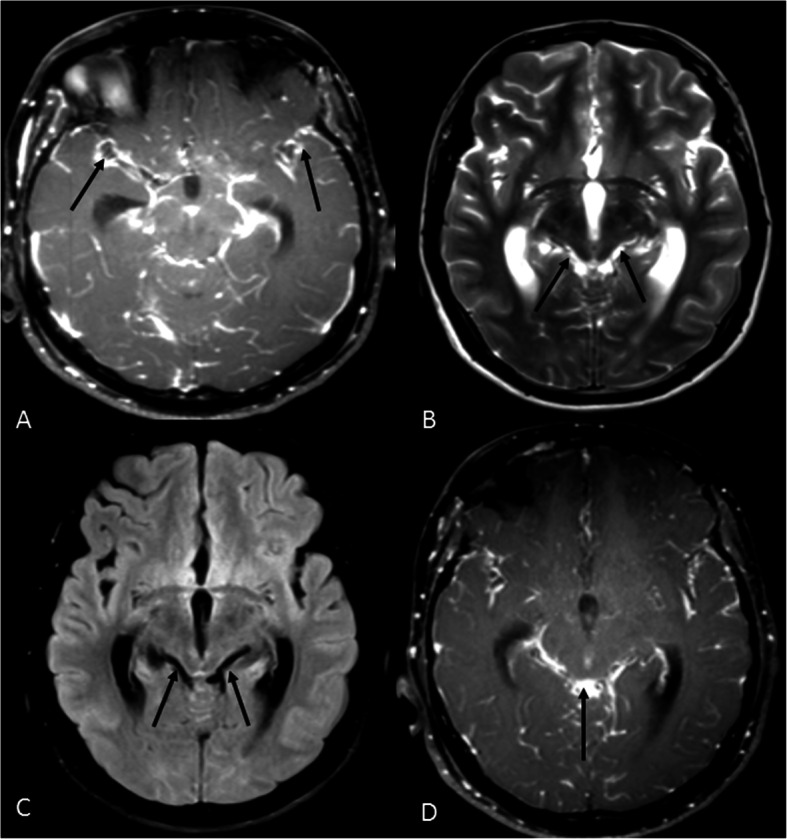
Magnetic resonance imaging of the brain at 2 months following the onset of symptoms. A Axial T1W post-gadolinium image of the brain at the level of the mid-brain shows nodularity of leptomeningeal enhancement. The black arrows point towards the nodular leptomeningeal enhancement along the Sylvian fissures. B T2W axial image of the brain at the level of the mid-brain shows expansion of the quadrigeminal cistern with inhomogeneous signal of the CSF. The black arrows are seen to point towards the inhomogeneous CSF signal in the quadrigeminal cistern. C Axial FLAIR image of the brain at the level of mid-brain shows complete signal suppression as compared with T2W image in B. The black arrows depict the suppression of CSF signal on FLAIR. D Post-contrast T1W axial images of the brain show enhancement along the CSF spaces corresponding to the T2 and FLAIR images. The black arrows point towards the enhancement along the CSF spaces
